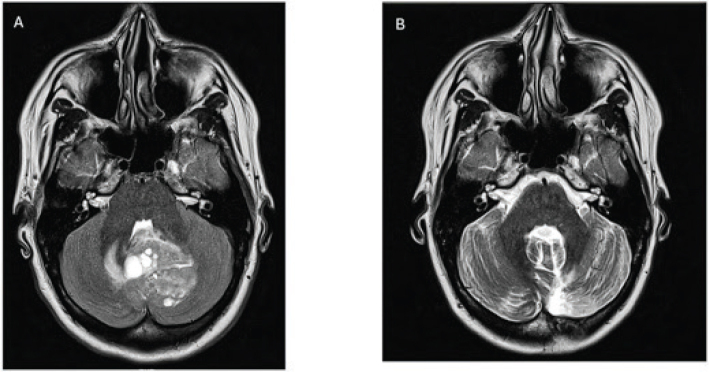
Brain magnetic resonance images. (A) In October 2013, preoperative MRI reveals a discreate mass in the cerebellum. (B) In February 2015, 16-month postoperative MRI scan shows the total resection of the tumor. MRI: magnetic resonance imaging.
